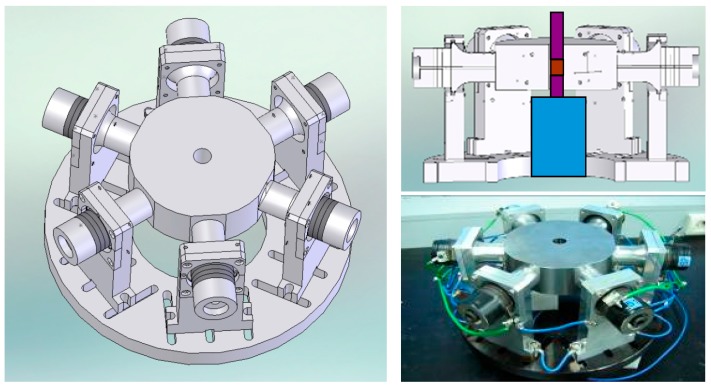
Assembled ultrasonic compaction mold system with supporting fixture.
